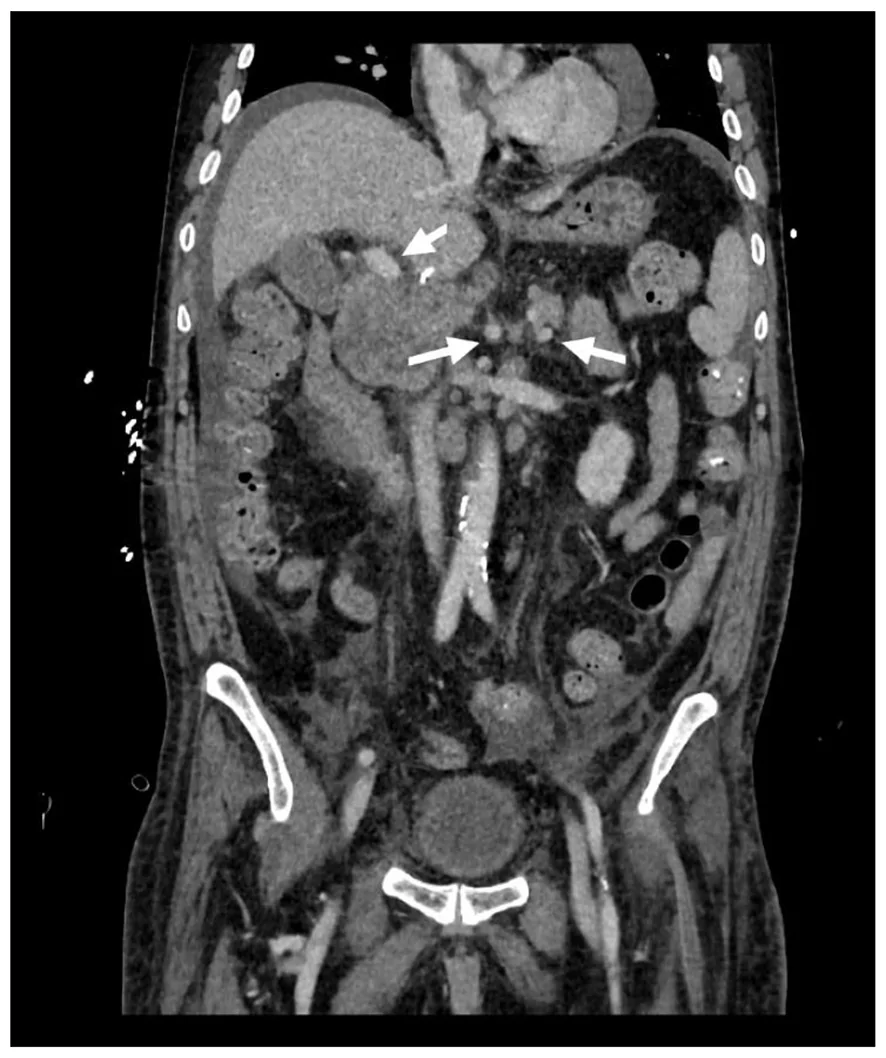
The figure shows the patient's abdominal CT, revealing multiple enlarged lymph nodes in the hepatic portal region and retroperitoneum.
